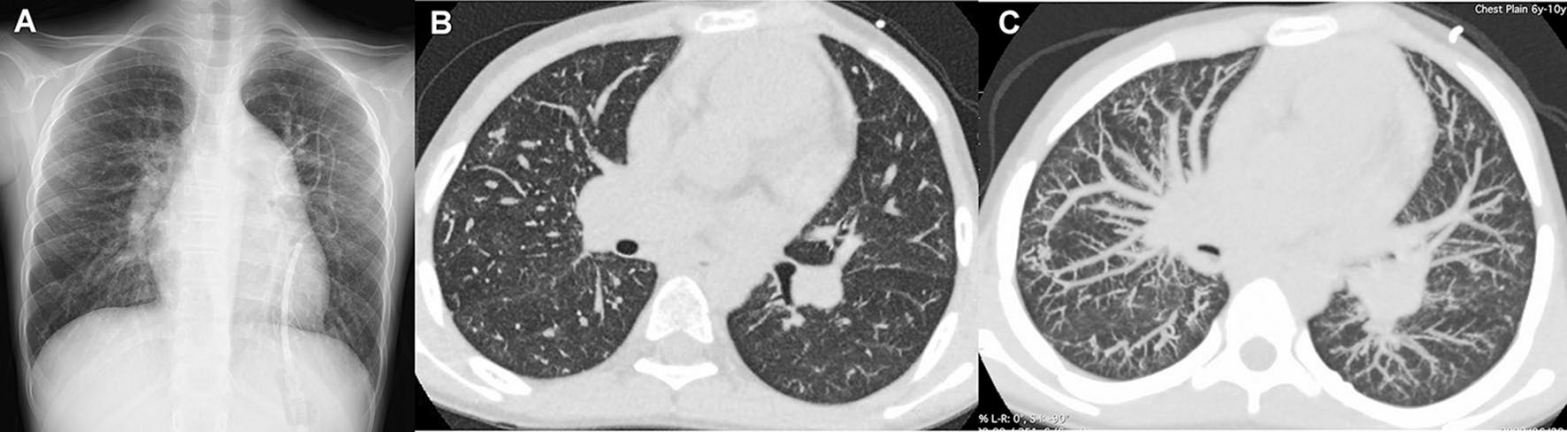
Hereditary hemorrhagic telangiectasia. An 8-year-old girl with decreased exercise tolerance. A CXR showed prominence of central and peripheral pulmonary vascular shadows. The PA segment was protruded. B CT revealed a number of nodular and tortuous shadows connected to the adjoining vasculatures, suggesting diffuse type AVMs. C MIP imaging provided better visualization of continuity of arteries, shunt vessels, and veins
